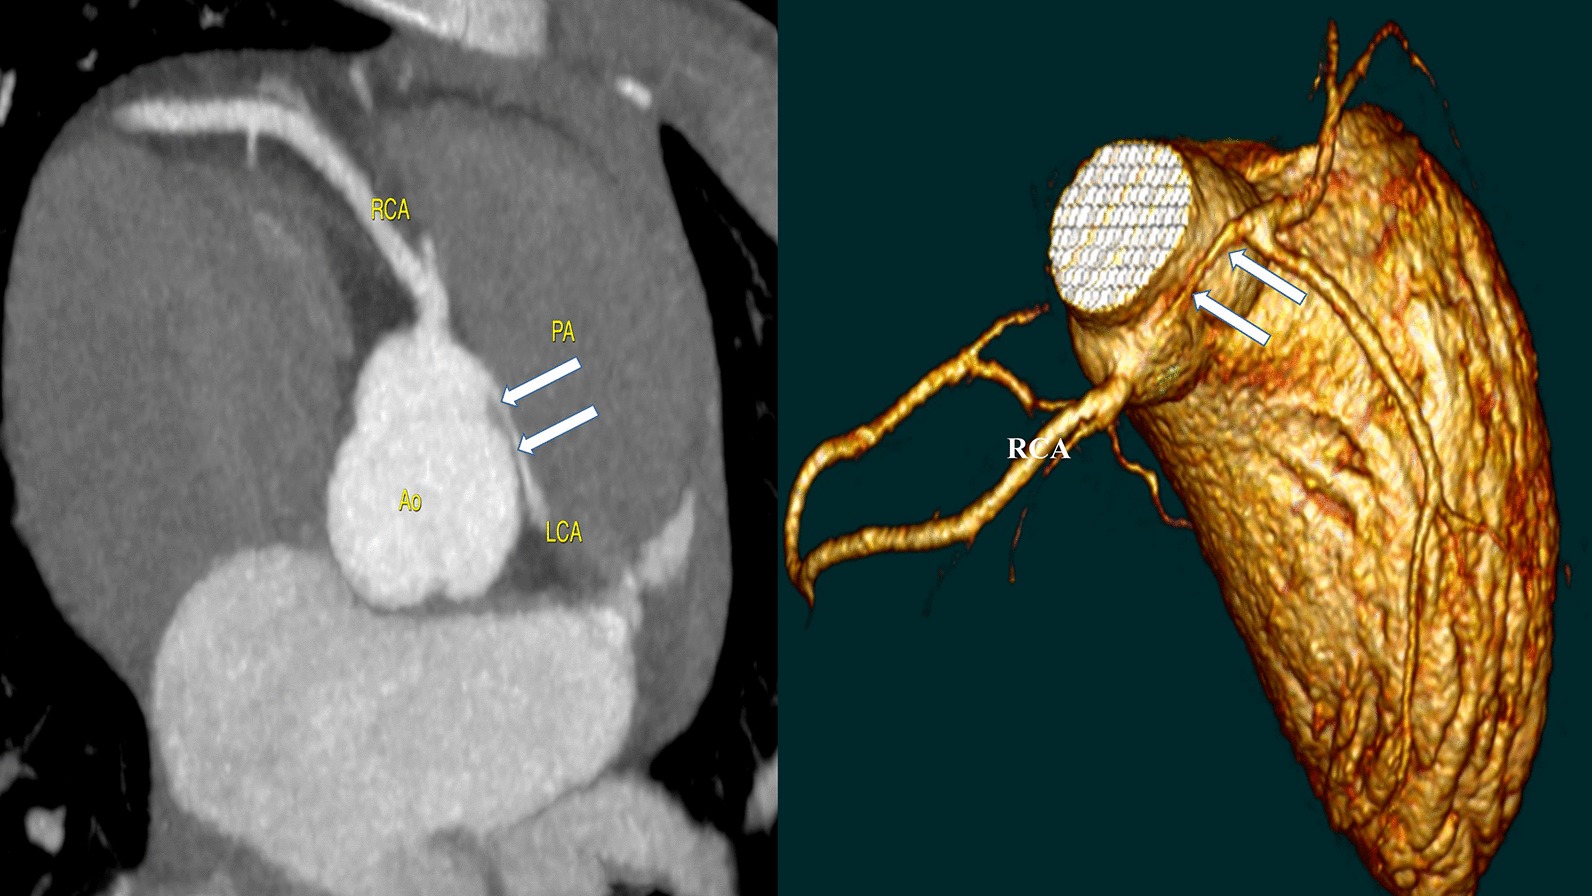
Computed tomography (CT) confirmed the diagnosis and showed the intramural course of the LCA with normal RCA, LAD and LCx. *LCA* left coronary artery, *RCA* right coronary artery, *LAD* left anterior descending artery, *LCx* left circumflex artery
